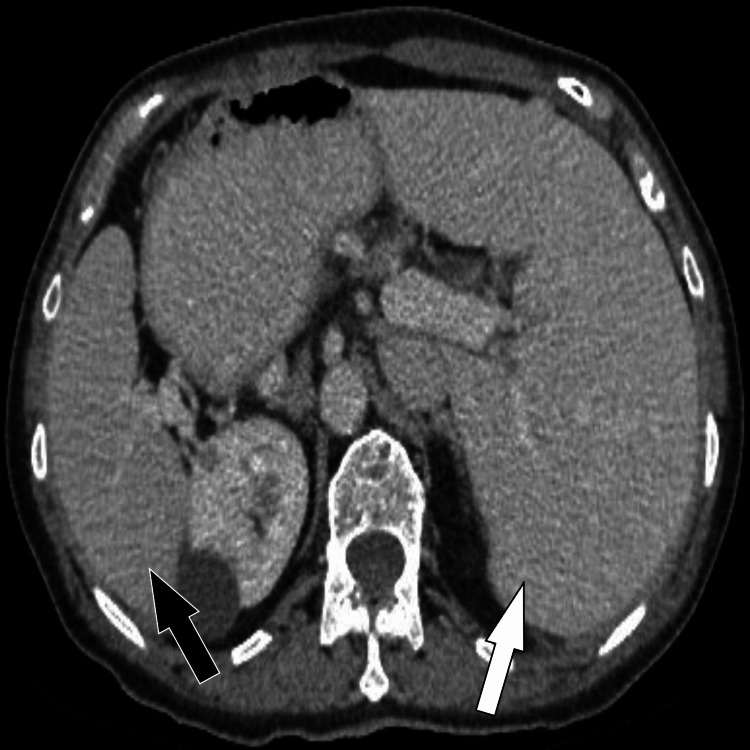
MSCT axial section (venous phase) through the upper part of the abdomen shows the liver on the left side (white arrow) and spleen on the right side (black arrow). MSCT: multi-slice computed tomography
